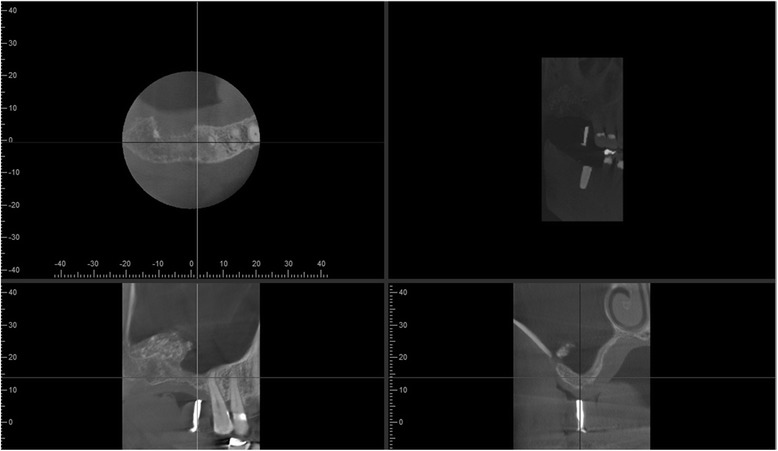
Right sinus about 12 months after first grafting procedure. Cone beam CT imaging shows little suitable bone at implant site, but grafted bone displaced distal to site. Bone hydroxyapatite particles were added as radiographic marker to the graft material for the first sinus augmentation procedure and are still visible as radiopaque specks
